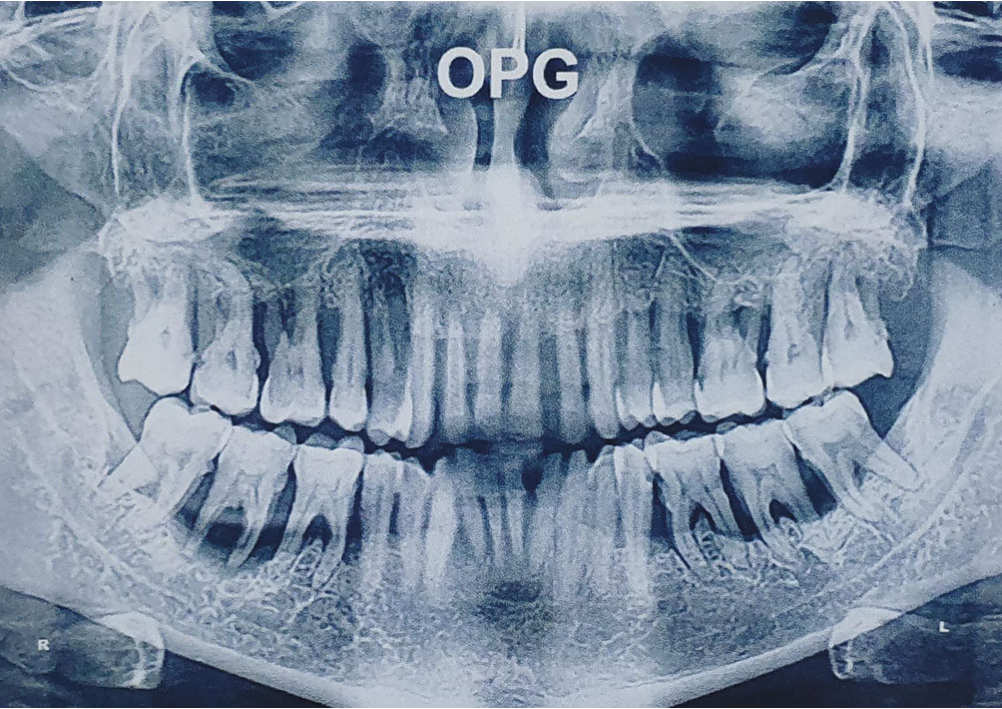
Orthopantomograph examination.
